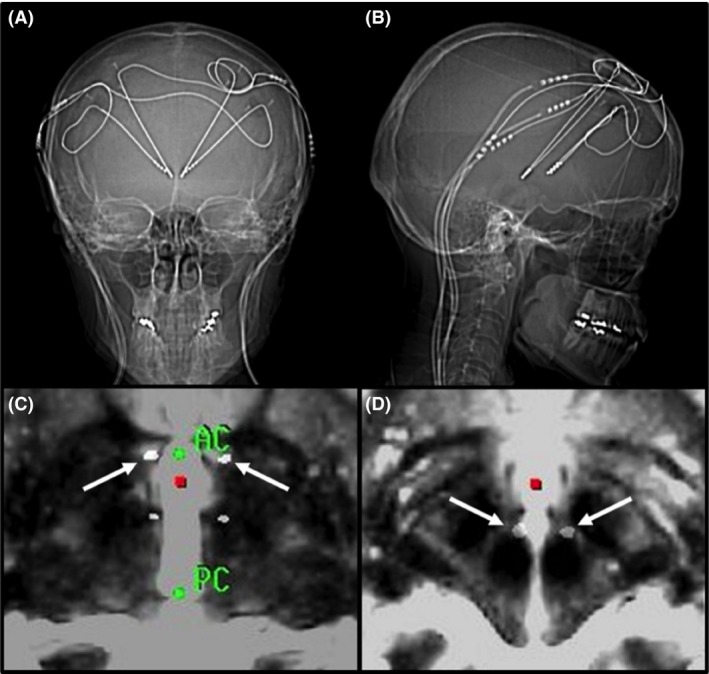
Postoperative scans demonstrating the location of the electrodes: (A) CT coronal view; (B) CT sagittal view; (C) CT fused with T2 MRI at AC‐PC‐level with arrows indicating the electrodes in the BNST; (D) CT fused with T2 MRI 4 mm below AC‐PC‐level with arrows indicating the electrodes in the MFB. CT, computed tomography; MRI, magnetic resonance imaging; AC, anterior commissure; PC, posterior commissure; BNST, bed nucleus of stria terminalis; MFB, medial forebrain bundle.
